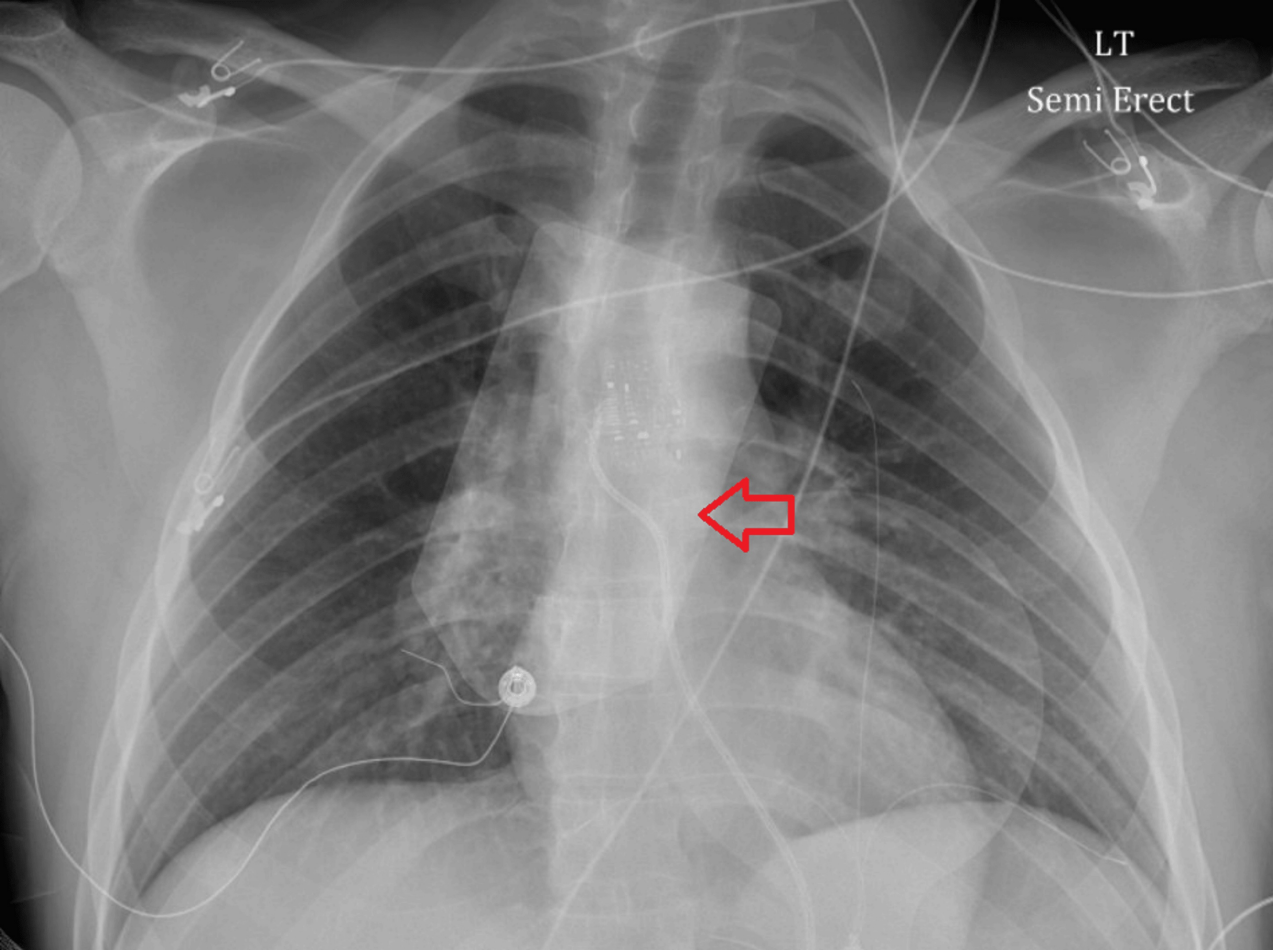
Initial chest X-ray not showing acute cardiopulmonary changes. Also shown with red arrow are the defibrillator pads placed after low ejection fraction was seen.
